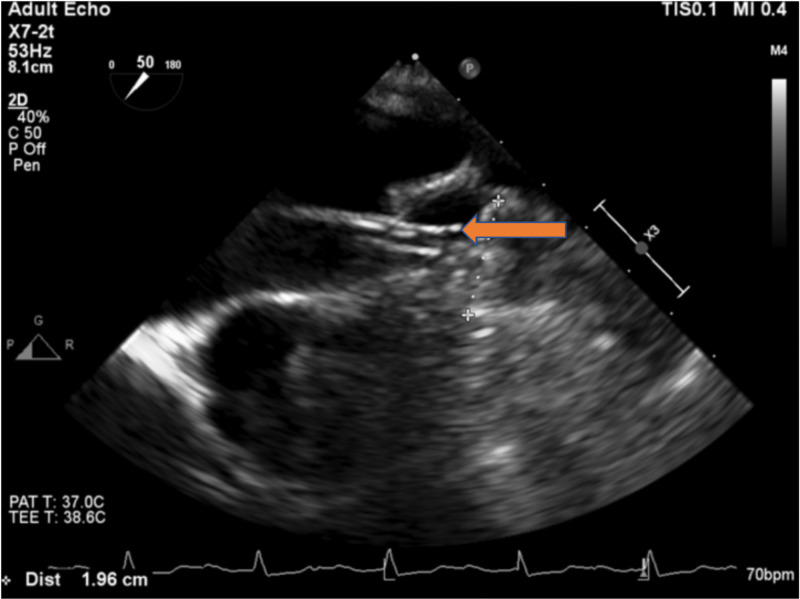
TEE showing a stable Watchman device without peri-device leak. TEE, transesophageal echocardiogram
